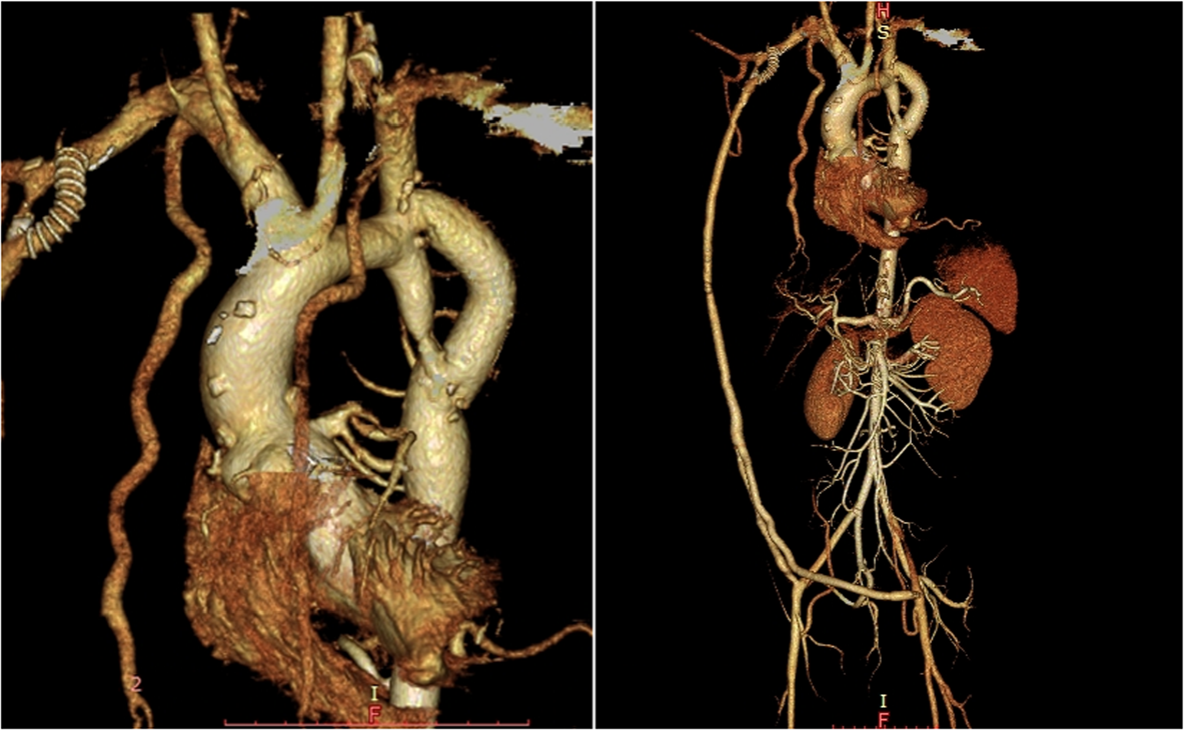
Postoperative multislice computed tomography angiography showed a patent graft
